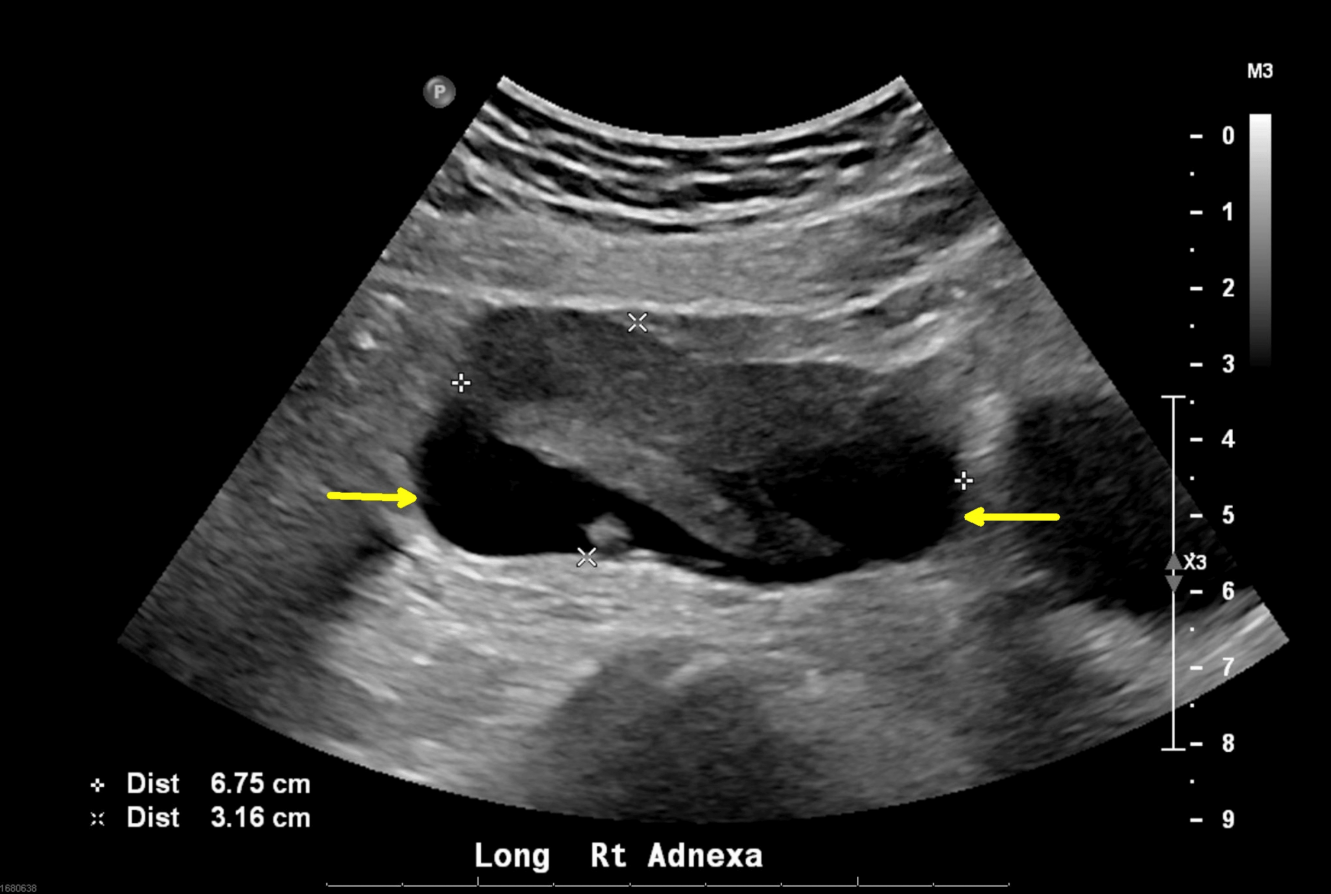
Pelvic ultrasound showing a 6.7 x 3.9 x 3.1 cm complex mass in the right adnexa with a tubular fluid component (between the yellow arrows).
